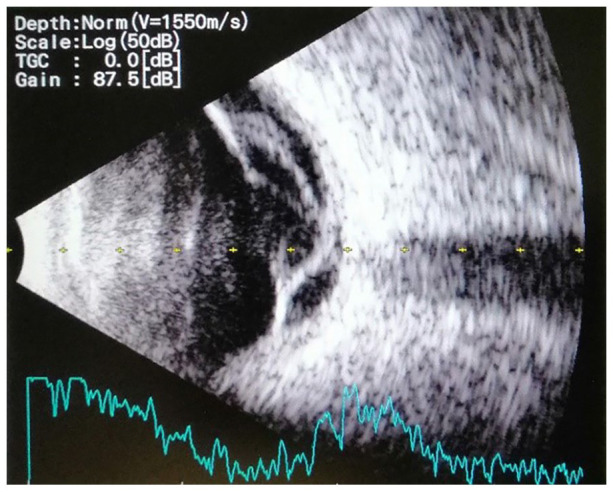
Right eye with tractional retinal detachment. Scan of B-ultrasonography of patients orbits.
